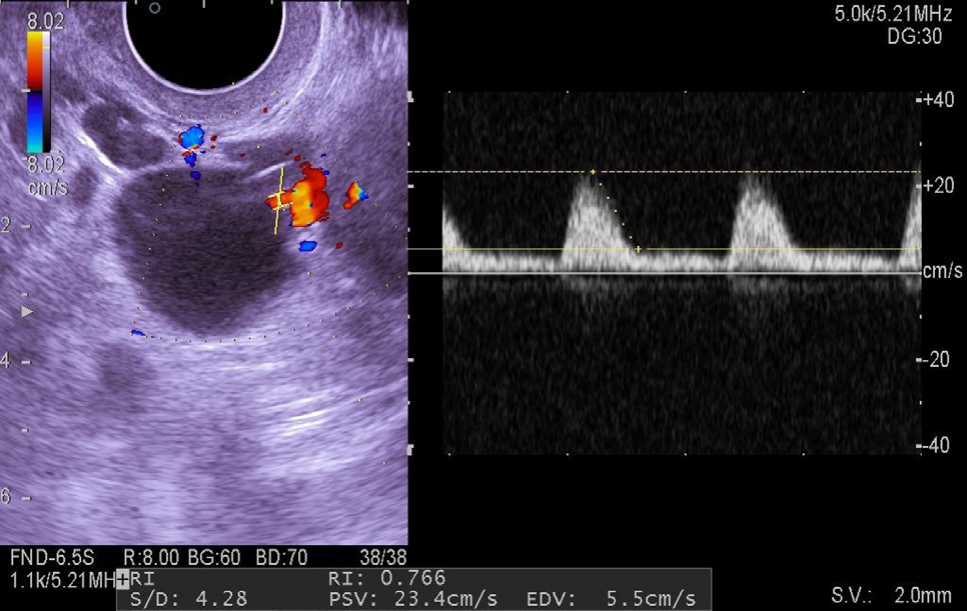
Sonogram of a follicular cyst with Doppler ultrasonography.
